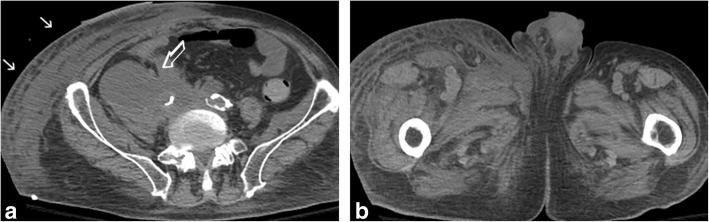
Non-contrast CT images through (a) the lower pelvis and (b) upper thighs demonstrating a large obstructing right pelvic tumor (open arrow), significant right-sided subcutaneous edema (arrows), and marked limb asymmetry due to right limb edema
